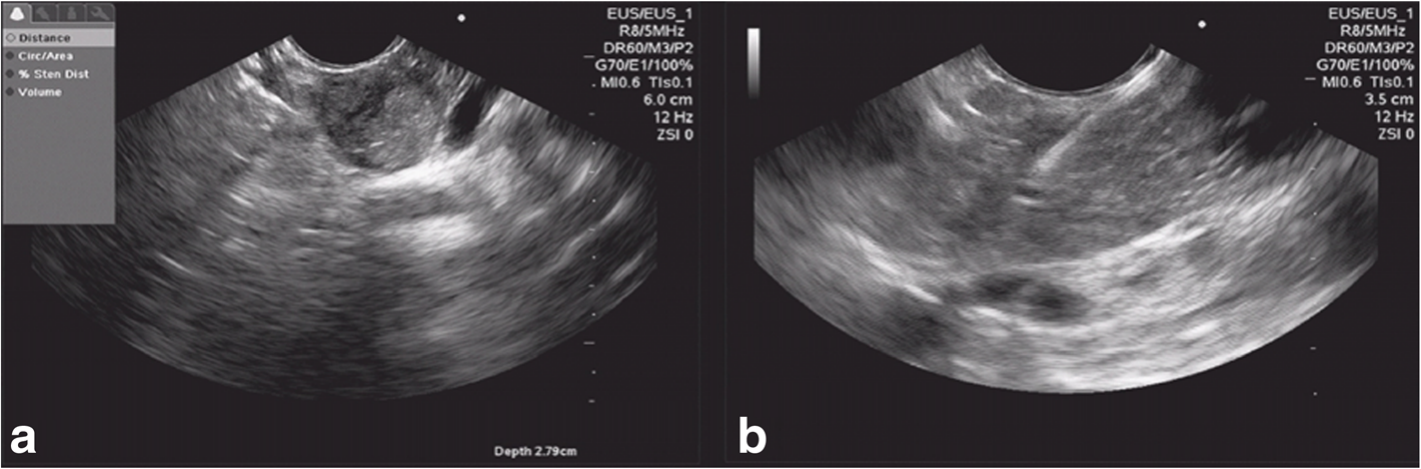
Endoscopic ultrasound view of the solid lesion with cystic areas in the pancreas. a Endoscopic ultrasound showed a regular, well-defined, homogeneous lesion with small anechoic (cystic) areas, measuring 2 × 2 cm, in the pancreatic body to neck transition. b Endoscopic ultrasound fine-needle aspiration with a 22 gauge needle
